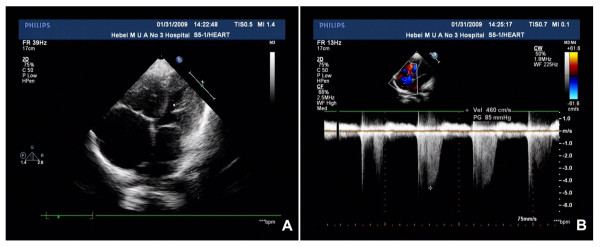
The color Doppler echocardiographic findings. A: M-mode and two-dimensional echocardiography shows the right atrial dimensions are 5.71×5.85 cm. B: Continuous wave Doppler shows the tricuspid valve regurgitation velocity is 4.6 m/s and the pressure gradient between right ventricle and right atrium is 85 mmHg.
